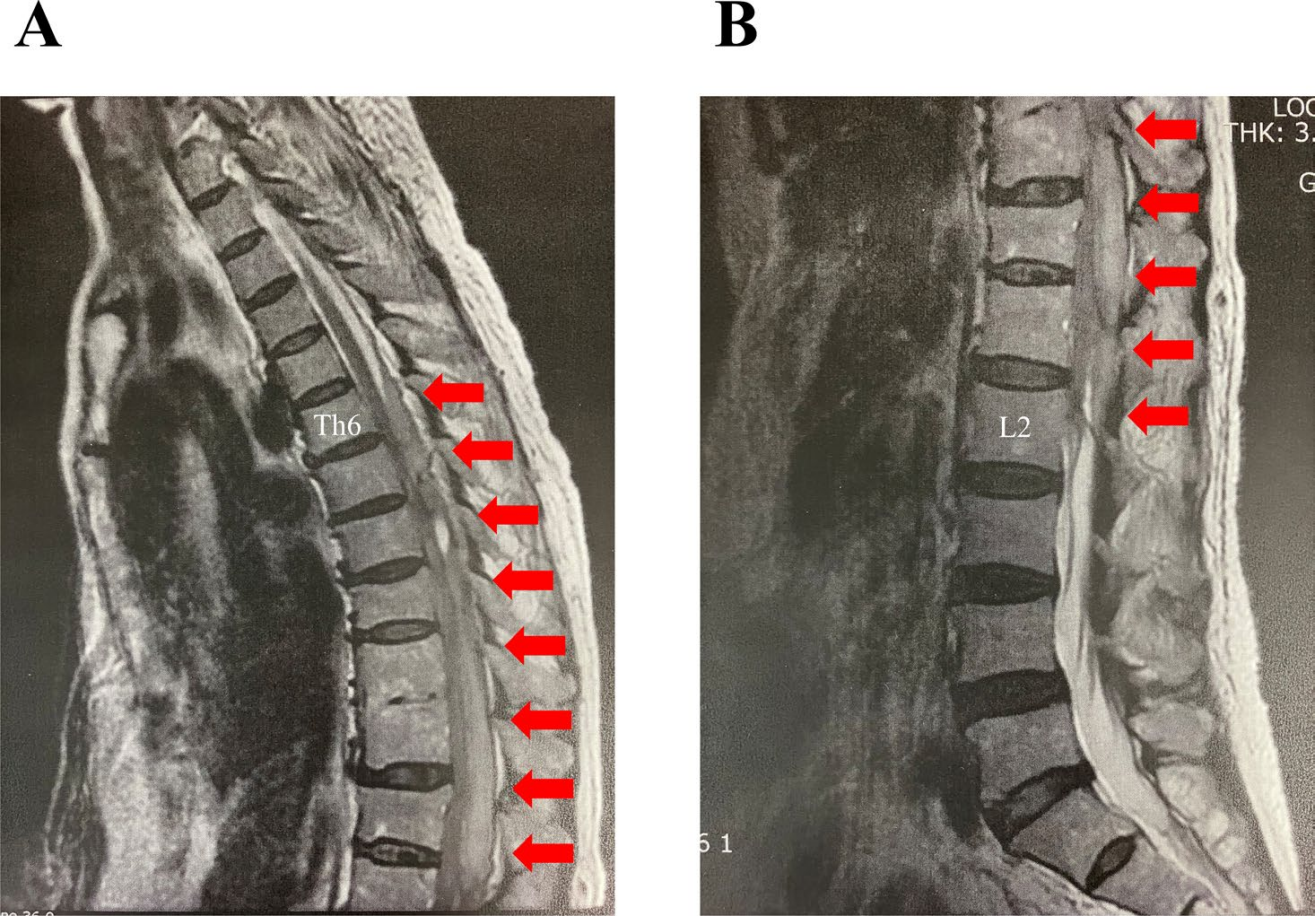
T2‐weighted sagittal magnetic resonance image. (A) Thoracic area; (B) lumbar area. Red arrows indicate spinal epidural hematoma from Th6 to L2.
